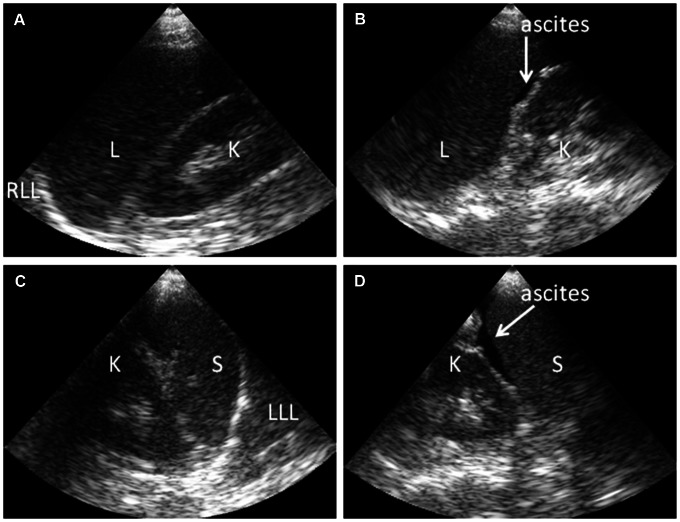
Ultrasonography findings showing presence or absence of ascites in dengue patients. Handheld ultrasonography performed at the bedside with the patient in supine position during examination. (A) Normal hepatic-renal area. (B) Minimal ascites in hepato-renal area (arrow). (C) Normal spleno-renal area. (D) Ascites in spleno-renal area (arrow). Abbreviations: L: liver; K: kidney, RLL: right lower lobe of lung; LLL: left lower lobe of lung; S: Spleen.
